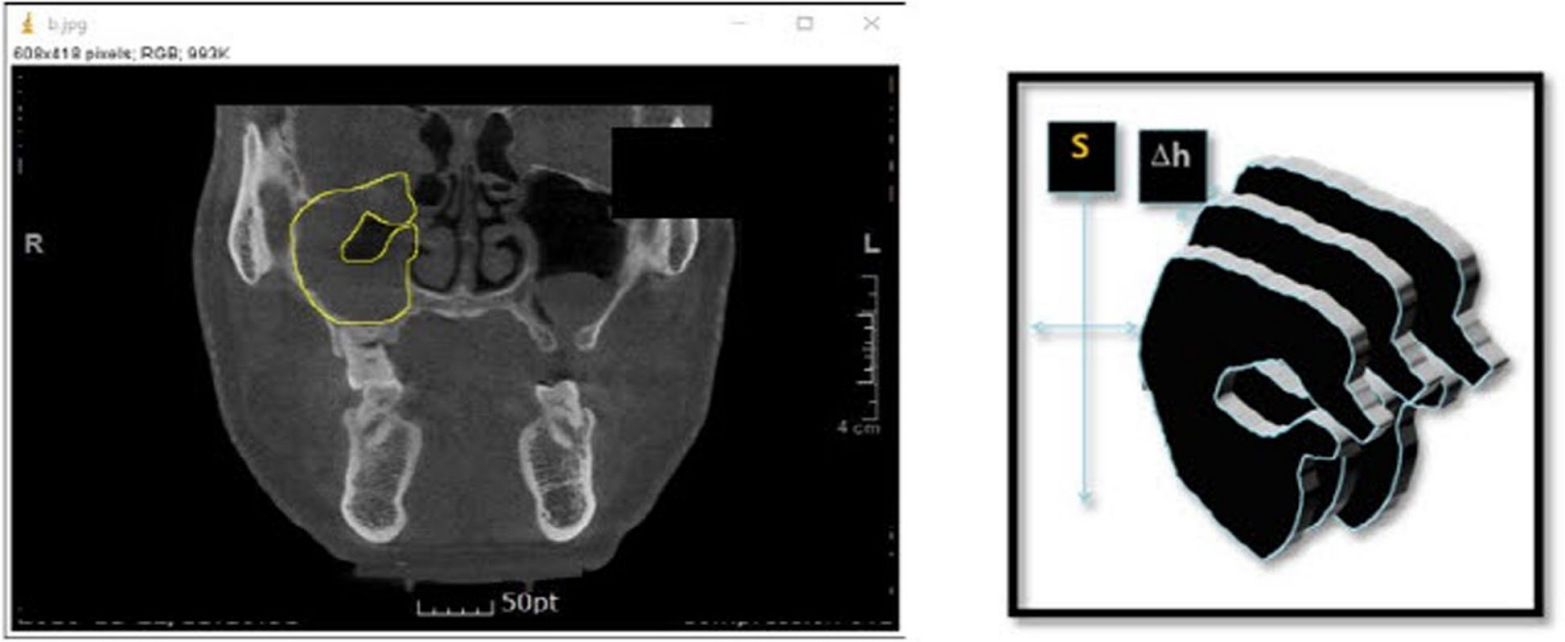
This figure shows the measurement of surface using ImageJ program (right) and the method to measure the volume of maxillary sinus using CT images (left)
